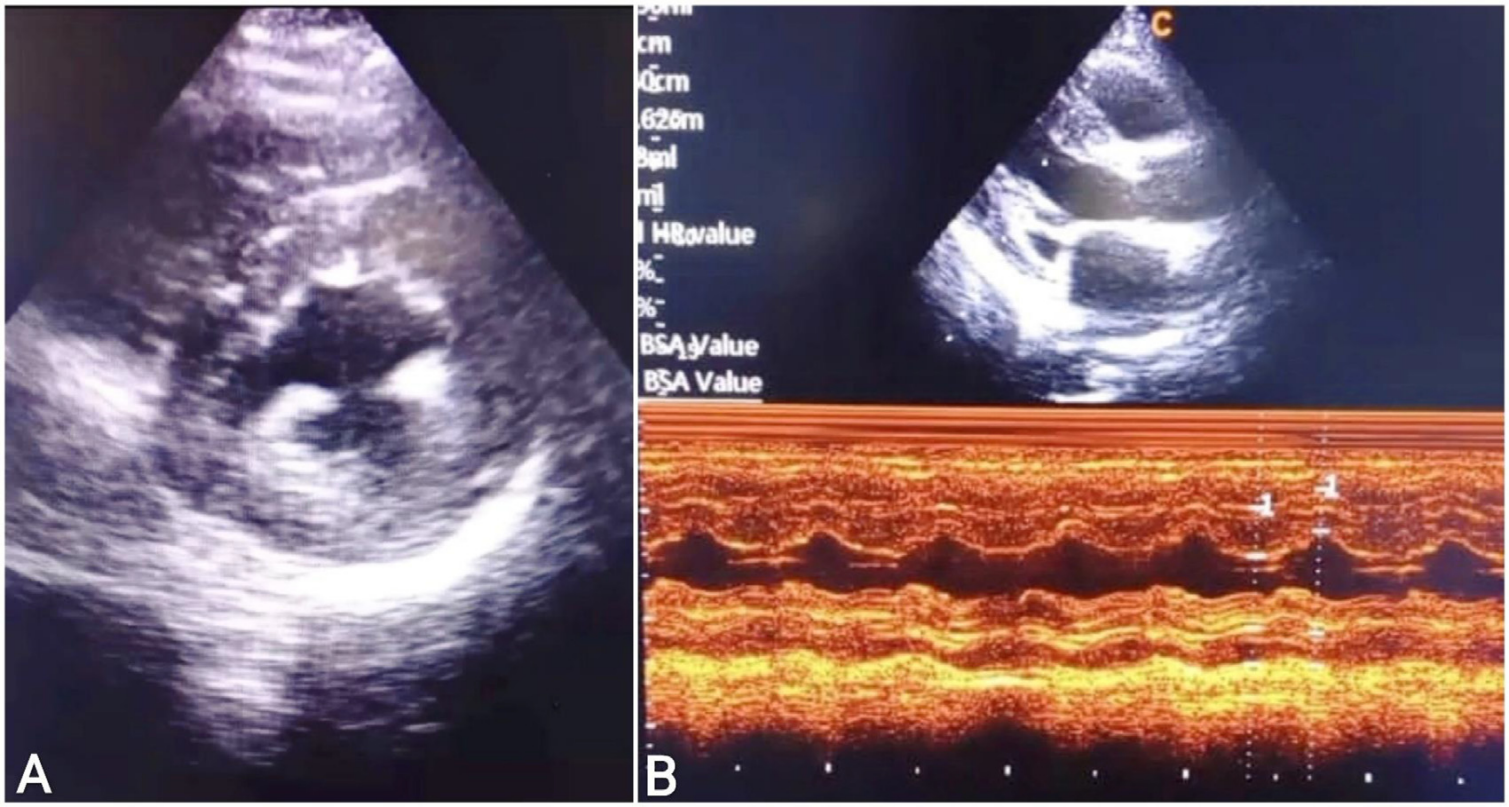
The two-dimensional transthoracic echocardiography: Parasternal short axis view (A) and parasternal long axis view (B) echocardiogram demonstrated left ventricular hypertrophy.
